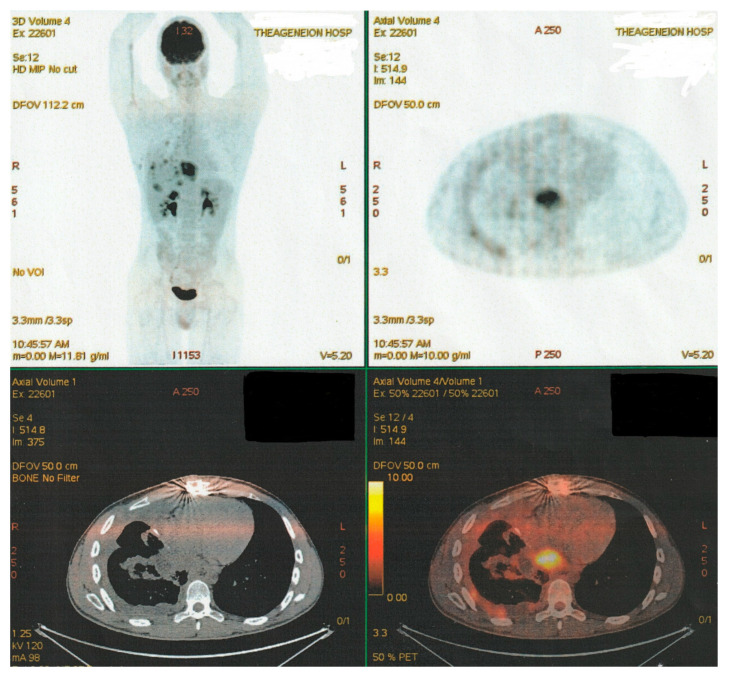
Latter PET-CT imaging control (after six “TIP-Regimen” sessions).
